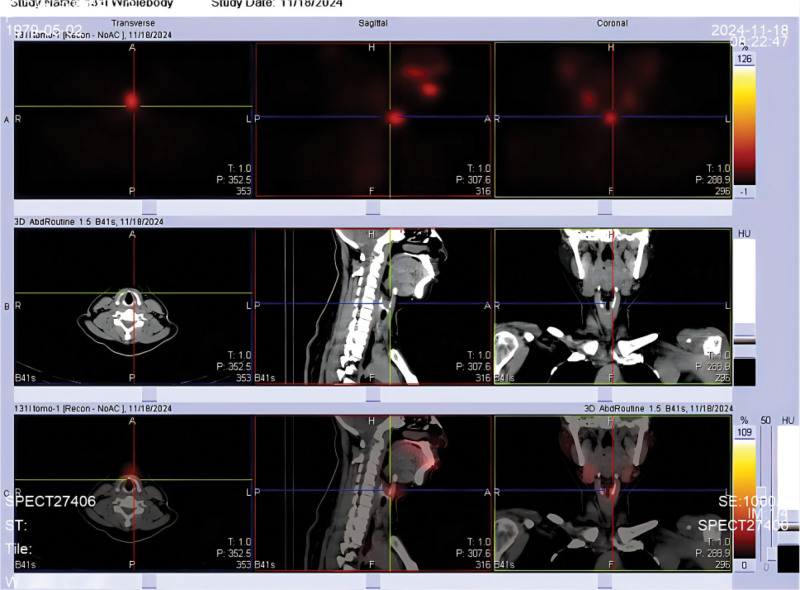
^131^I SPECT/CT fusion imaging at 48 hours localizes the uptake focus to the midline just inferior to the hyoid bone (crosshair), and the corresponding low-dose CT shows no discrete soft-tissue mass, consistent with a thyroglossal duct remnant. SPECT/CT = single photon emission computed tomography, computed tomography.
